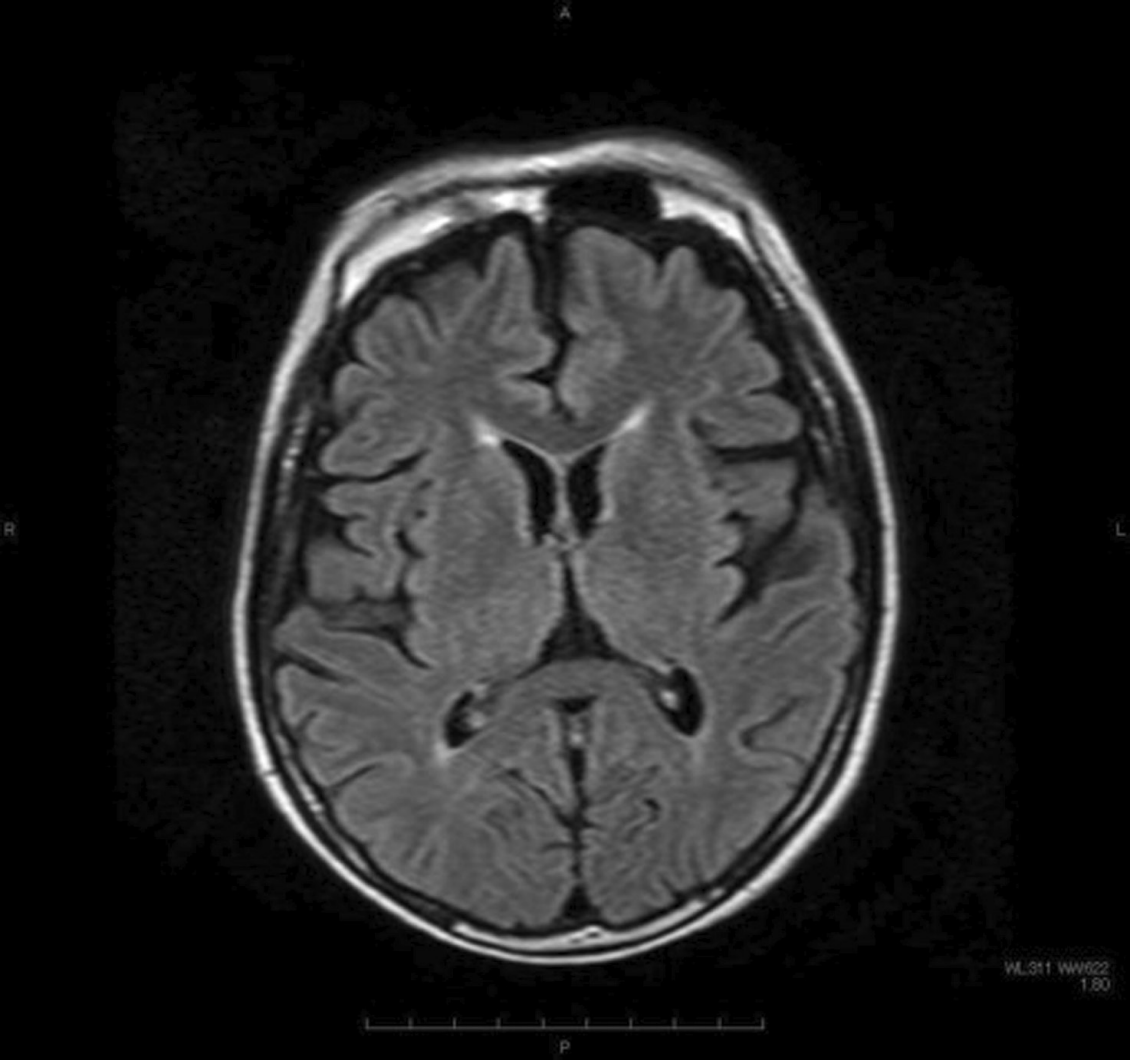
Brain magnetic resonance imaging findings. Brain magnetic resonance imaging on admission showed brain atrophy, but no abnormal findings in thalamus.
